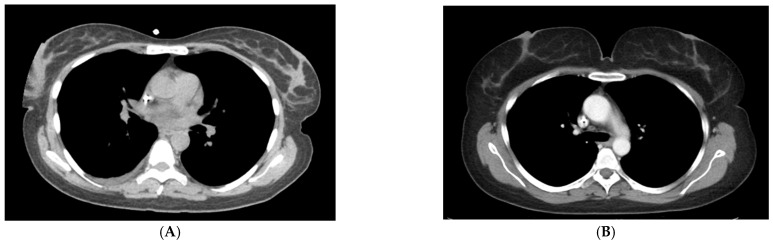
Same patient without and then with bra in place. In the first image (A), a portion of the right breast is not imaged due to collimation. In the second image (B), both breasts are positioned in the area of reduced current due to the wearing of a bra during the CT scan.
